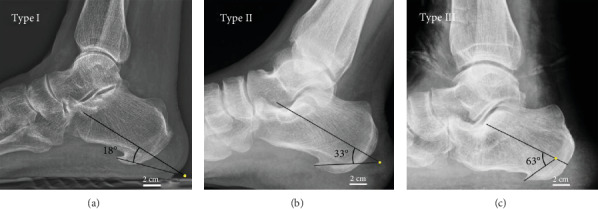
X-rays of classification of PCS. Type I, angle was less than 30° (a). Type II, angle was from 30° to 60° (b). Type III, angle was more than 60° (c).
